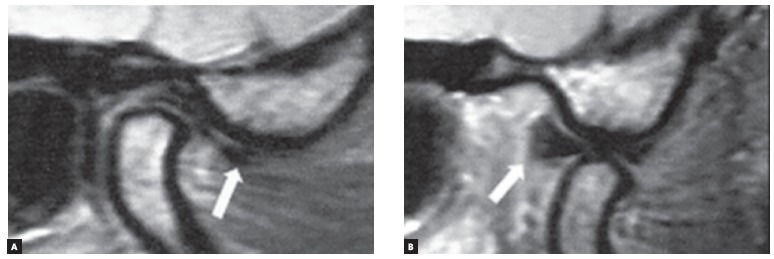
A) MRI sagittal slice of the right TMJ with the mouth closed, evincing
